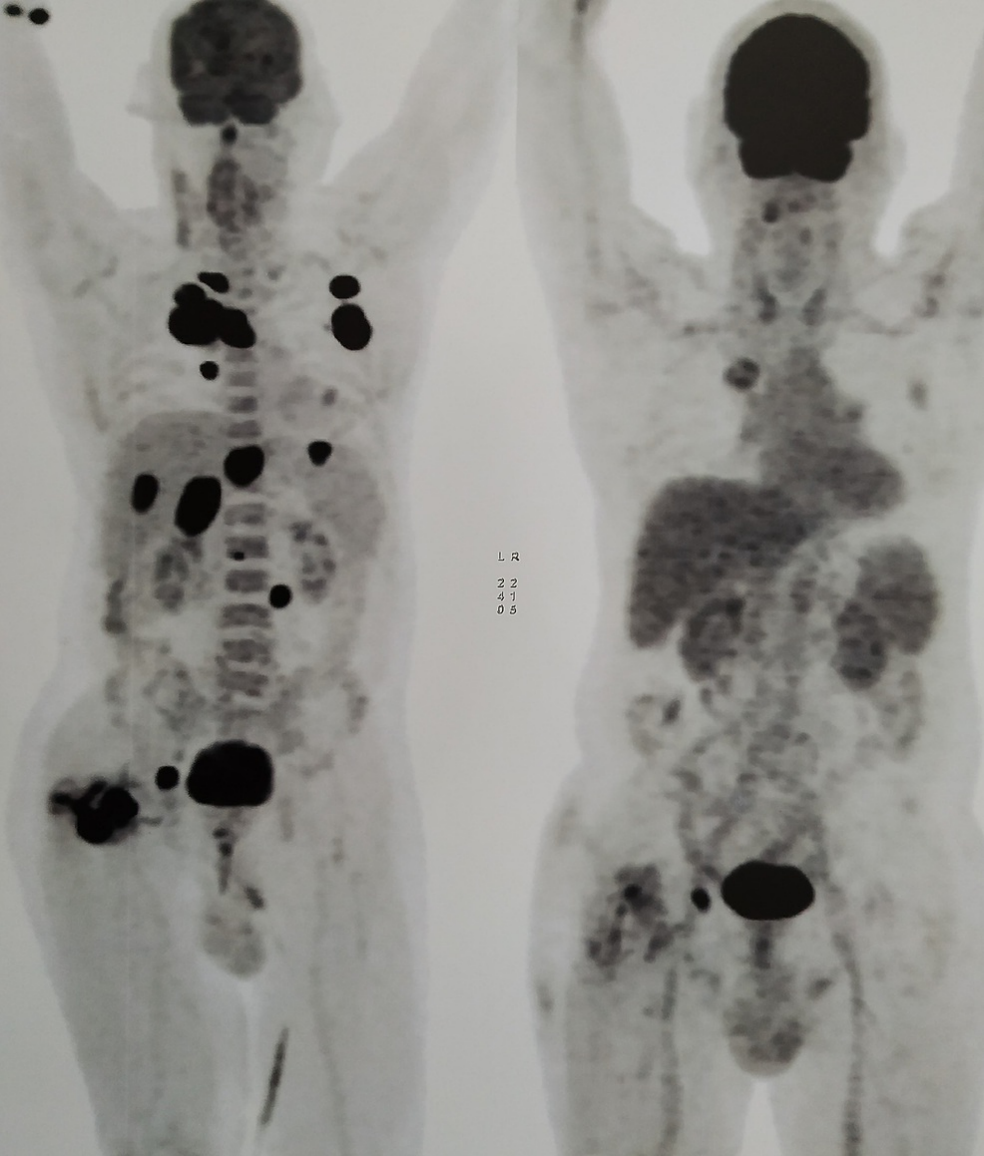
Interim whole-body PET-CT scan after four cycles of pembrolizumab showing near-complete response compared to baseline PET-CT. PET-CT, positron emission tomography-computed tomography.
